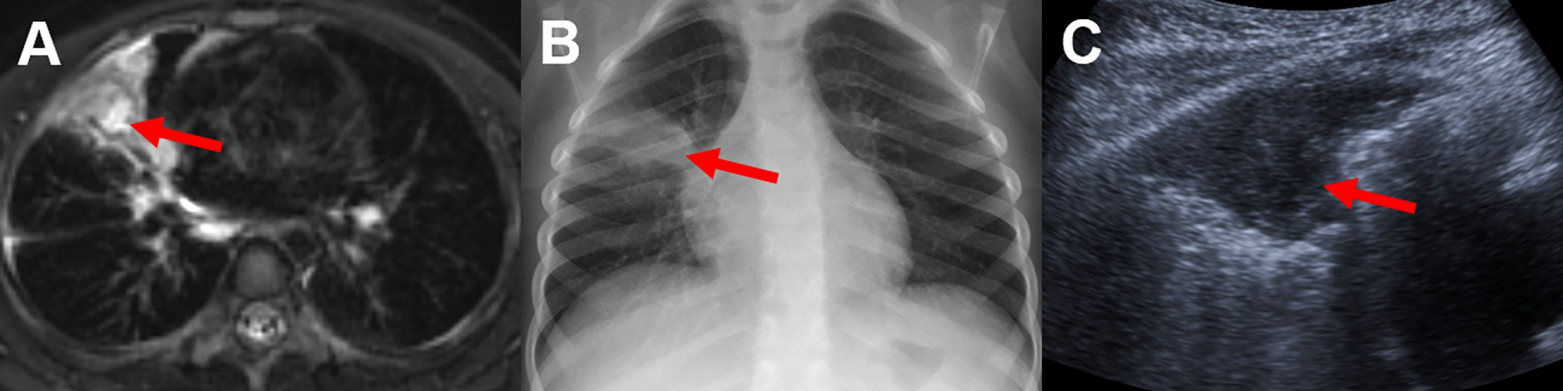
Contrast-enhanced MRI, CXR and LUS study of a 3-year old child. T2 weighted MRI (A) demonstrates an abscess formation within the consolidation in the middle lobe (red arrow), not detected by CXR (B) showing only consolidation (red arrow). In LUS (C) the abscess demarcates barley and was not diagnosed initially (red arrow).
